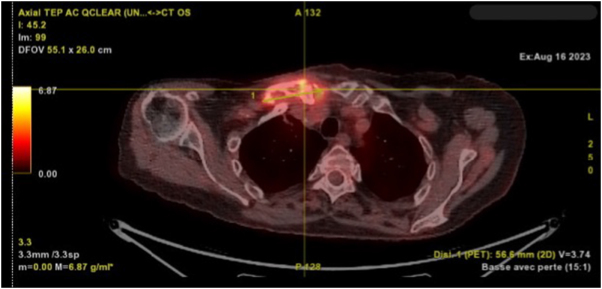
PET CT showing lytic osteoarticular lesion at the right sternocostoclavicular junction, measuring approximately 56 mm. *Printed with permission; copyrights retained by Elise Lupon, MD, PhD.*
